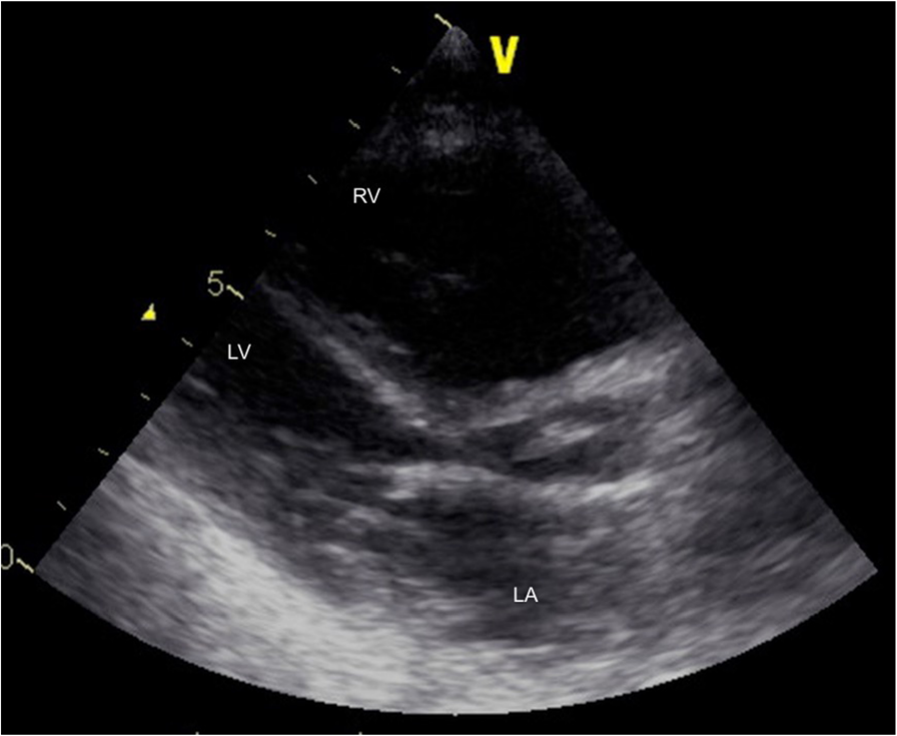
Echocardiogram in the parasternal long axis view showing the enlargement of the right-side heart and the thickening of the mitral valve leaflets. RV, right ventricle; LV, left ventricle; LA, left atrium
